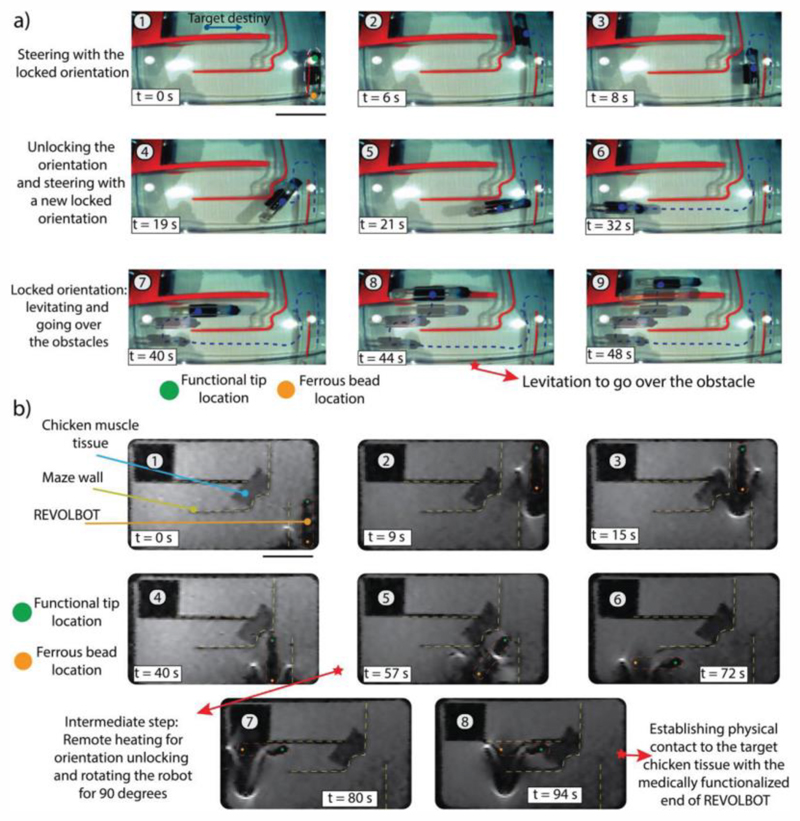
Navigation and steering demonstration of the REVOLBOT prototype using the proposed locking/unlocking mechanism in a synthetic maze while monitoring it by a visual camera and MR scan images. a) Top-view visual camera image snapshots of the robot: translating up and down in a locked state,^[1–3]^ unlocking and changing its planar orientation and translating in the new locked orientation to left and up directions,^[4–6]^ and overlaid video snapshot images going over an obstacle in 3D by levitating the robot using the out-of-plane magnetic pulling forces.^[7–9]^ b) Top-view MR scan image snapshots of the robot: translating up and down in a locked state,^[1–4]^ unlocking and changing its planar orientation and translating in the new locked orientation to left and up directions,^[5–7]^ and contacting a chicken tissue in snapshot 8. Here, the robot tip (green colored dot) and the environment can be monitored by MR images during the operation.
